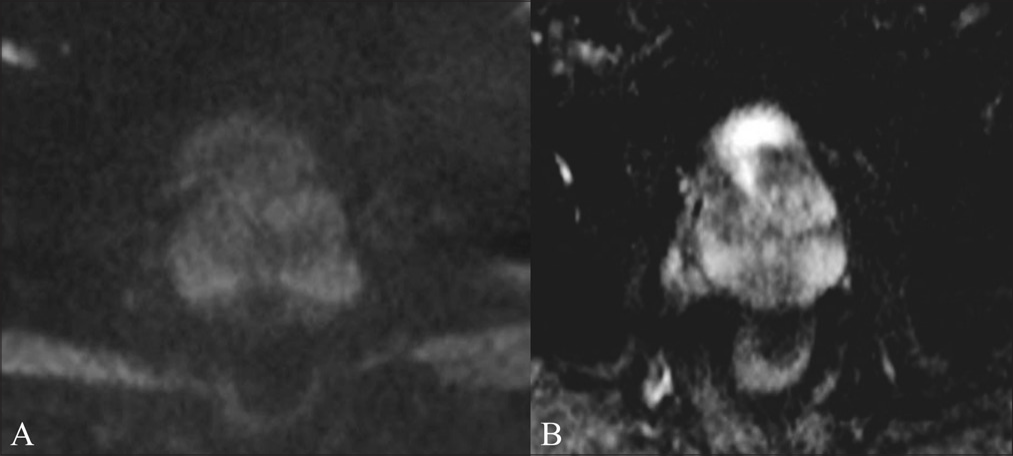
Follow up images of case 2 after 4 months. DWI (b=800) (A) shows resolution of the previously shown lesion. Normalization of signal is also seen in the respective ADC map (B)
